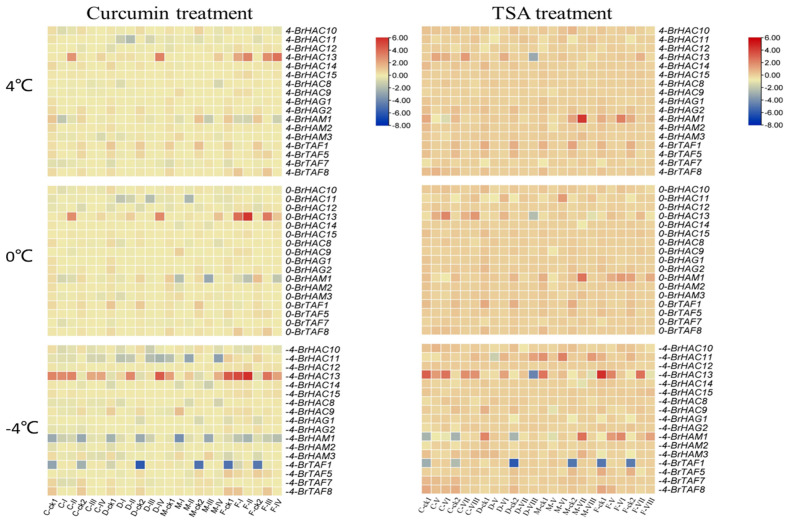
Expression analysis of *BrHATs* in leaves after inhibitor treatment. “C” is “CT-2360”, “M” is “MXW-1”, “D” is “DT-9”, and “F” is “2018-FJT”. ck1: 12 h; ck2: 24 h; I: 40 µmol/L curcumin spray treatment for 12 h; II: 40 µmol/L curcumin spray treatment for 24 h; III: 90 µmol/L curcumin spray treatment for 12 h; IV: 90 µmol/L curcumin spray treatment for 24 h; V: 50 µmol/L TSA spray treatment for 12 h; VI: 50 µmol/L TSA spray treatment for 24 h; VII: 100 µmol/L TSA spray treatment for 12 h; VIII: 100 µmol/L TSA spray treatment for 24 h.
